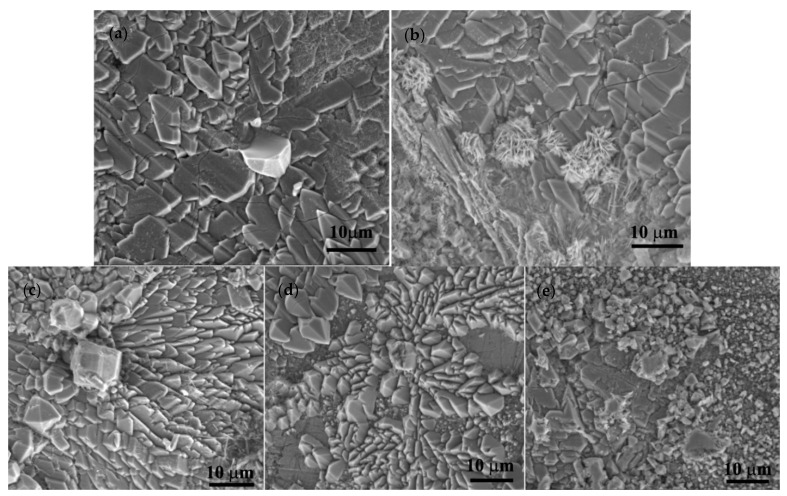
SEM after immersion period of three days and ultrasound cleaning: (a) Zn, (b) Zn_3_Mg, (c) ZnMg_0.4_Y, (d) ZnMg_0.5_Y, and (e) ZnMg_0.6_Y.
